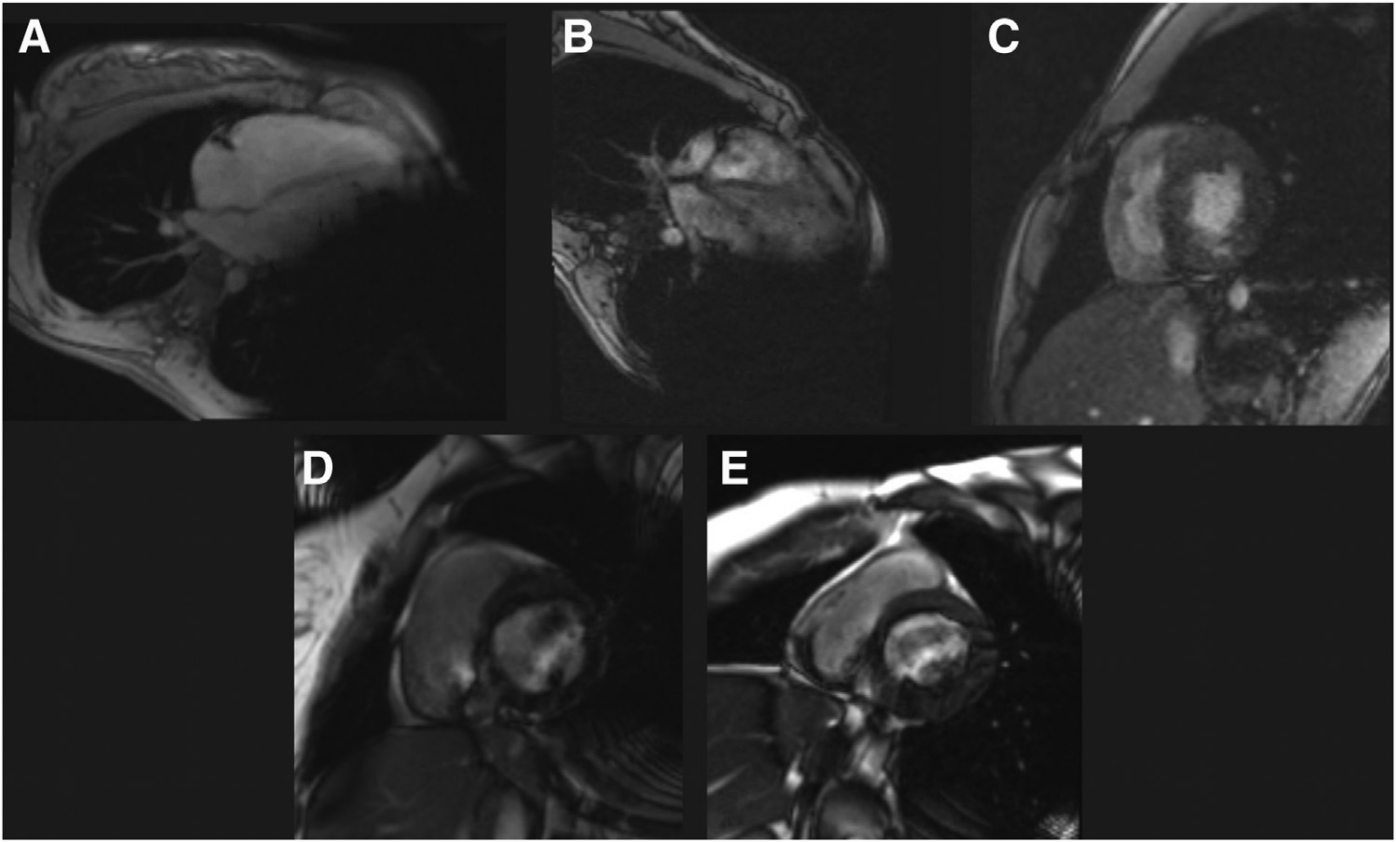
Referred sample cardiac magnetic resonance images for each patient from Table 1.
